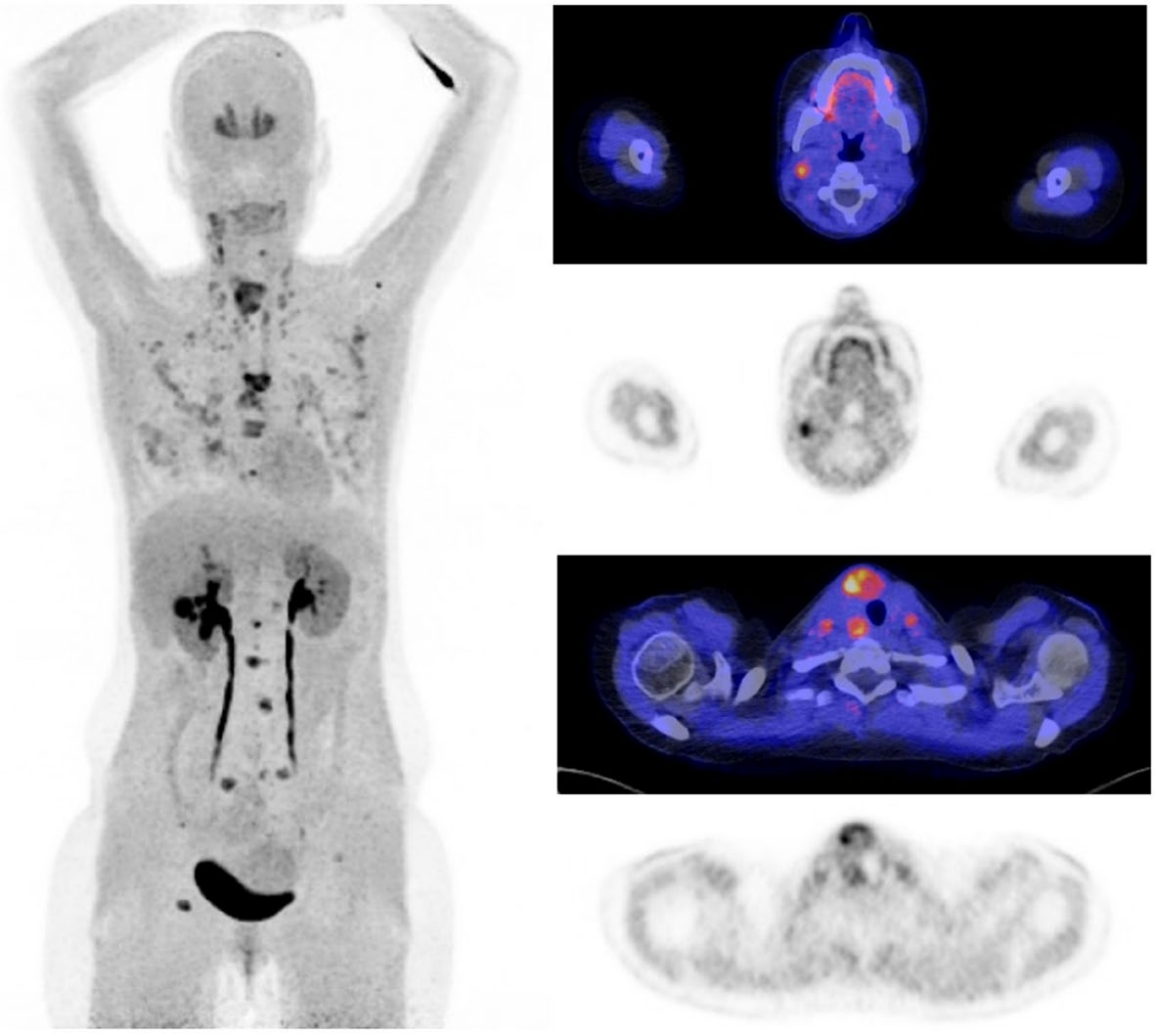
Images of an ^18^F-DOPA PET/CT performed preoperatively in a patient with medullary thyroid cancer with locoregional lymph node metastases. In addition, the ^18^F-DOPA PET/CT identified distant metastatic lesions in both lungs and bone
